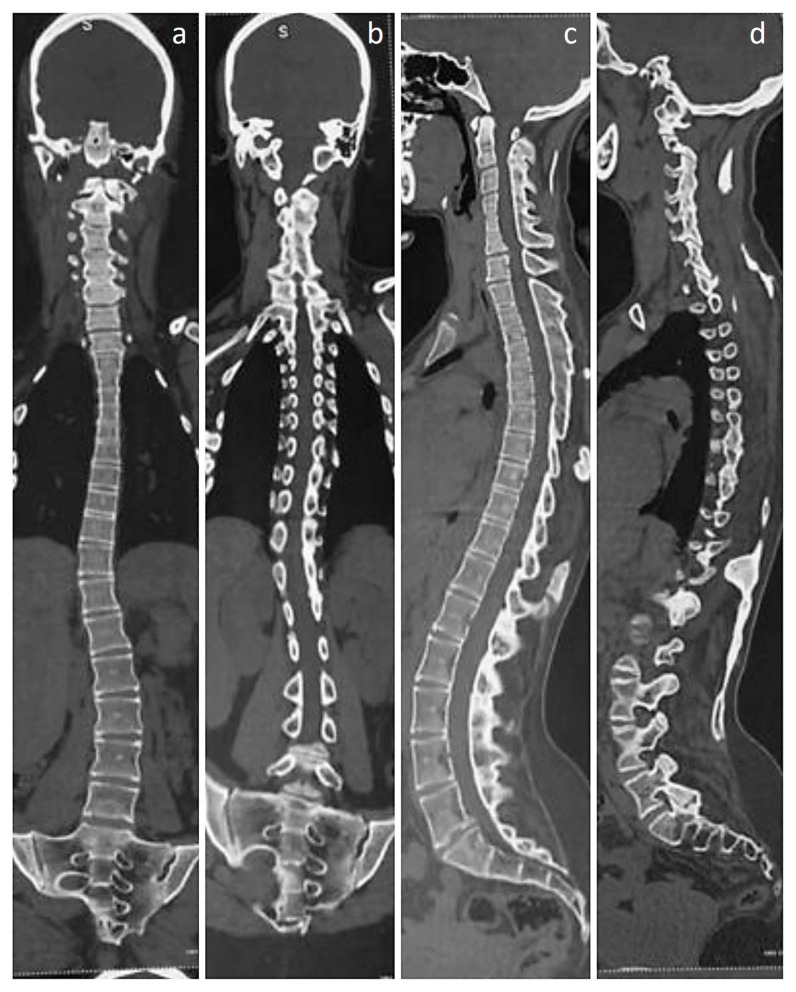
Whole-spine CT scans, both coronal and sagittal sections. (a–d) Sections clearly show the thoracolumbar scoliosis, along with fused cervical disc spaces and posterior elements, with only the discs above and below C7 appearing to be spared. (c) Sagittal section shows the fusion of thoracic disc spaces and the thoracolumbar posterior elements. (c,d) The heterotopic bone involving the posterior muscles are also seen clearly in the sagittal views.
